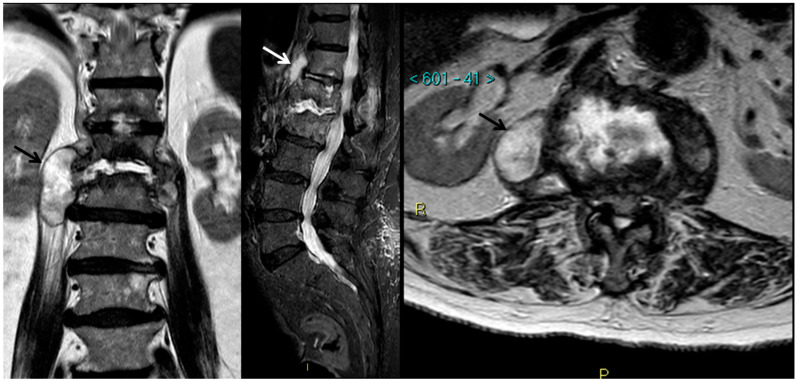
Tuberculous spondylodiscitis (T12-L2) in an 84-year-old woman. MRI detects bone and disks involvement together with several voluminous paravertebral abscesses (arrows).
